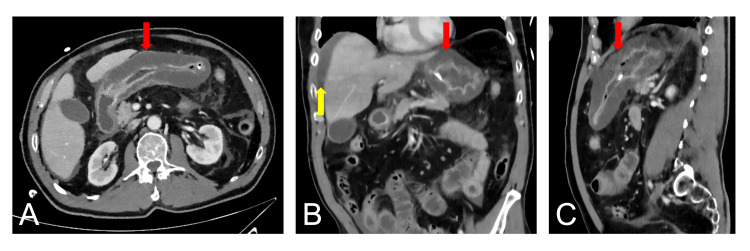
Initial imaging on admission Abdominal contrast-enhanced computed tomography scan on the day of admission revealed a circumferential thickening of the gastric wall (marked with a red arrow) and free abdominal fluid (marked with a yellow arrow). (A) Axial plane. (B) Coronal plane. (C) Sagittal plane.
